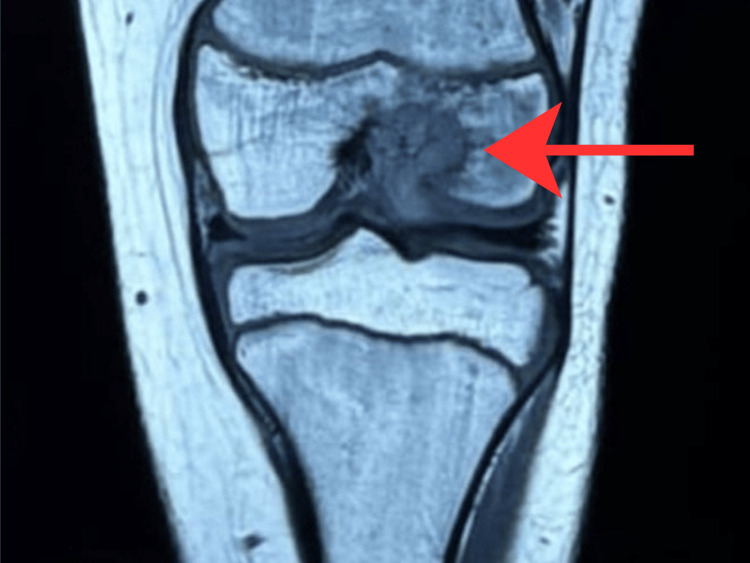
MRI of the left knee. An osseous neoplastic lesion (red arrow) located at the medial aspect of the lateral femoral condyle, extending to the adjoining intercondylar roof with intra-articular involvement, suggestive of osteoblastoma. Minimal interstitial edema was noted in the anterior cruciate ligament, with fibers remaining intact. MRI, magnetic resonance imaging
